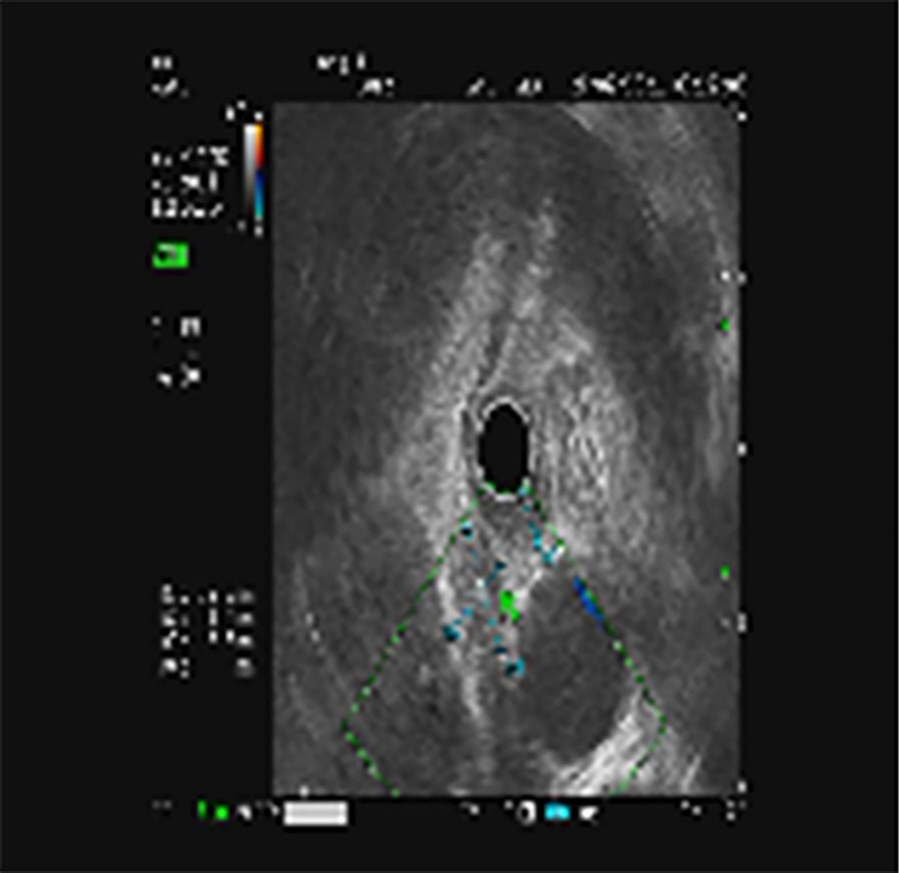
Endoscopic ultrasonography: mixed echo lesions in the hepatogastric space: combined with the history, foreign body wrapping may occur
